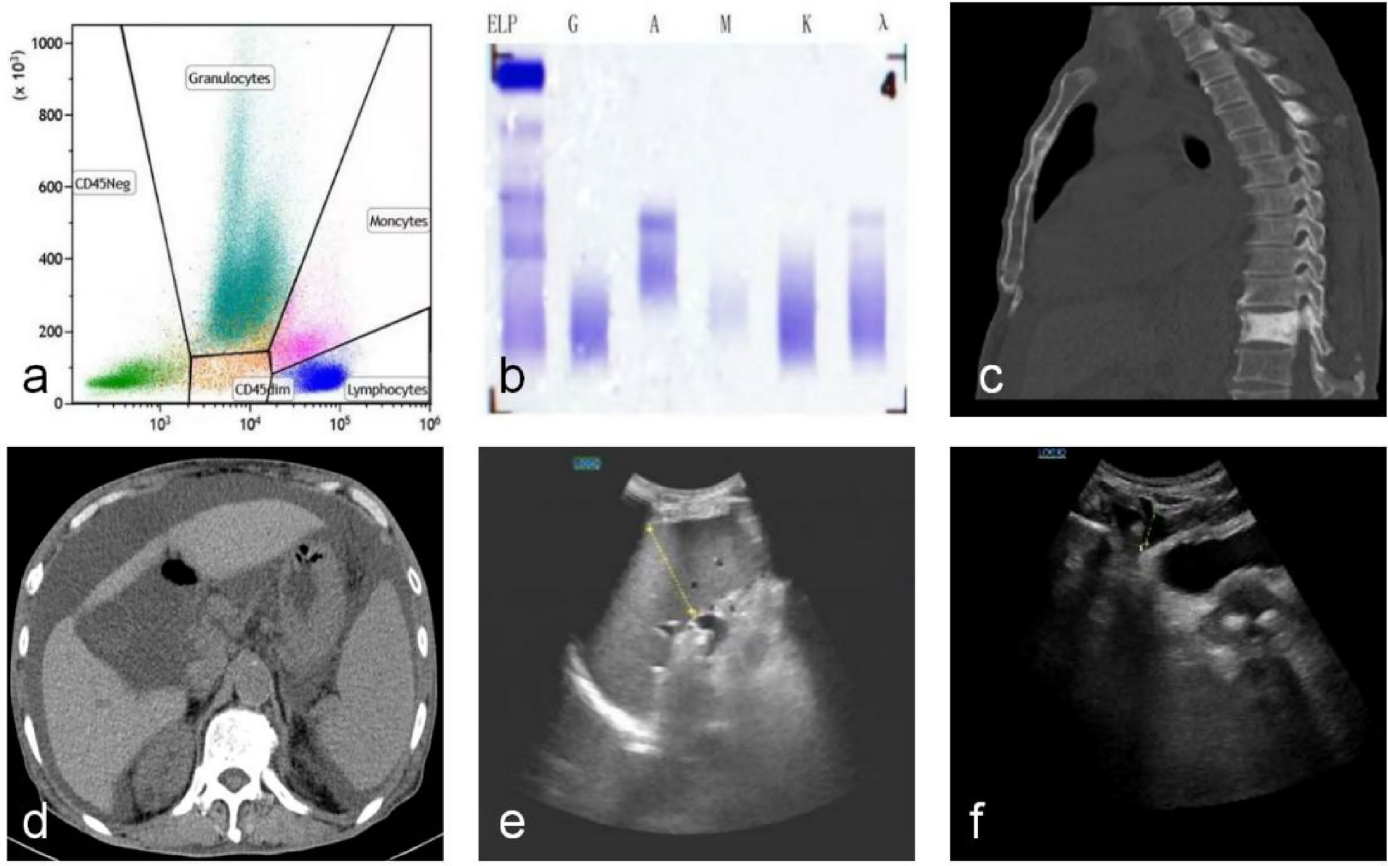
The examination results of the patient before and after treatment are as follows. (a) FCM detected approximately 0.11% of monoclonal plasma cells. (b) Analysis of serum immunofixation electrophoresis (IF) typing results: The type of monoclonal immunoglobulin is IgA-*λ*. (c) Pre-Treatment Thoracoabdominal CT: Patchy Hyperdense Shadows in the Cervical Spine and Multiple Thoracolumbar Vertebrae. (d) Pre-Treatment Abdominal CT: Large Amount of Fluid Accumulation in the Pelvic and Abdominal Cavities; Splenomegaly. (e) Post-Treatment Abdominal Ultrasound: Mild Splenomegaly. (f) Post-Treatment Ascites Ultrasound Examination: Fluid Accumulation in the Intestinal Spaces of the Lower Abdomen.
